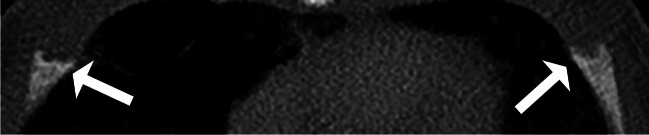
Symmetric bilateral costochondral junction spurs in a 3-week-old boy evaluated with chest computerized tomography (CT) due to respiratory distress. Axial CT with 2.5-mm slice thickness shows bilateral costochondral junction spurs with central concavity at the level of the 4th ribs (*arrows*)
